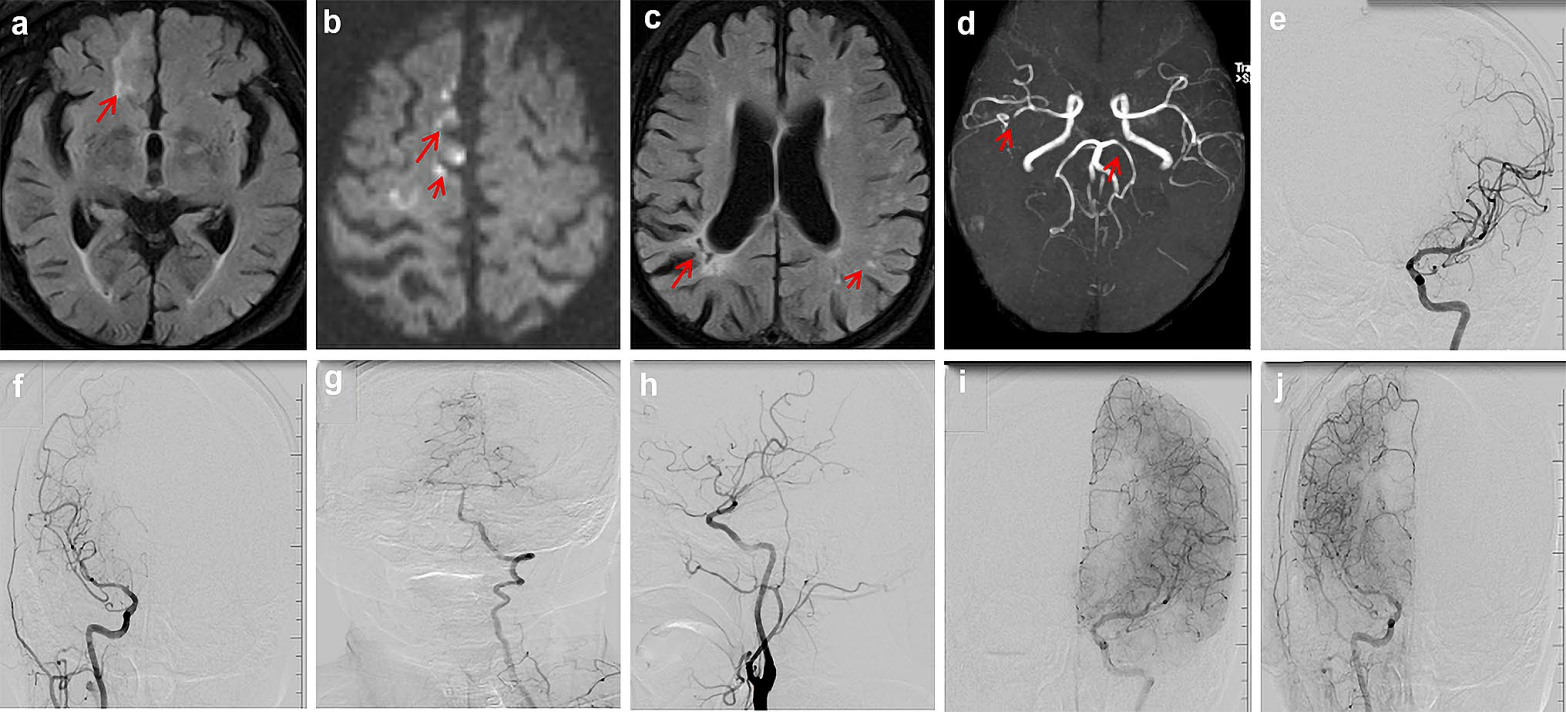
a, b Flair sequence and diffusion-weighted imaging show old ischemic white matter lesions (a, arrow) and multiple acute punctate infarctions (b, arrows), respectively, in the right frontal lobe. c Flair sequence shows an old watershed infarct in the right junction of the temporal and occipital lobes (long arrow) and multiple left-sided subcortical punctate ischemic demyelinating lesions (short arrow). d Brain MRA shows the bilateral absence of the ACA, right MCA M2 segment mild stenosis (arrow), and left PCA P1 segment mild stenosis (arrow). e, f, g Frontal DSA shows the absence of the left (e) and right (f) ACAs, and the PCA compensates for the ACA through the pial artery in the late arterial phase (g). h Lateral DSA shows the absence of the right ACA. i, j DSA shows that the left MCA compensates for the left ACA through the pial artery (i), while the right MCA compensates for the right ACA through the pial artery (j). *ACA* anterior cerebral artery, *CT* computed tomography, *DSA* digital subtraction angiography, *Flair* fluid attenuated inversion recovery, *MRA* magnetic resonance angiography, *MCA* middle cerebral artery, *PCA* posterior cerebral artery
